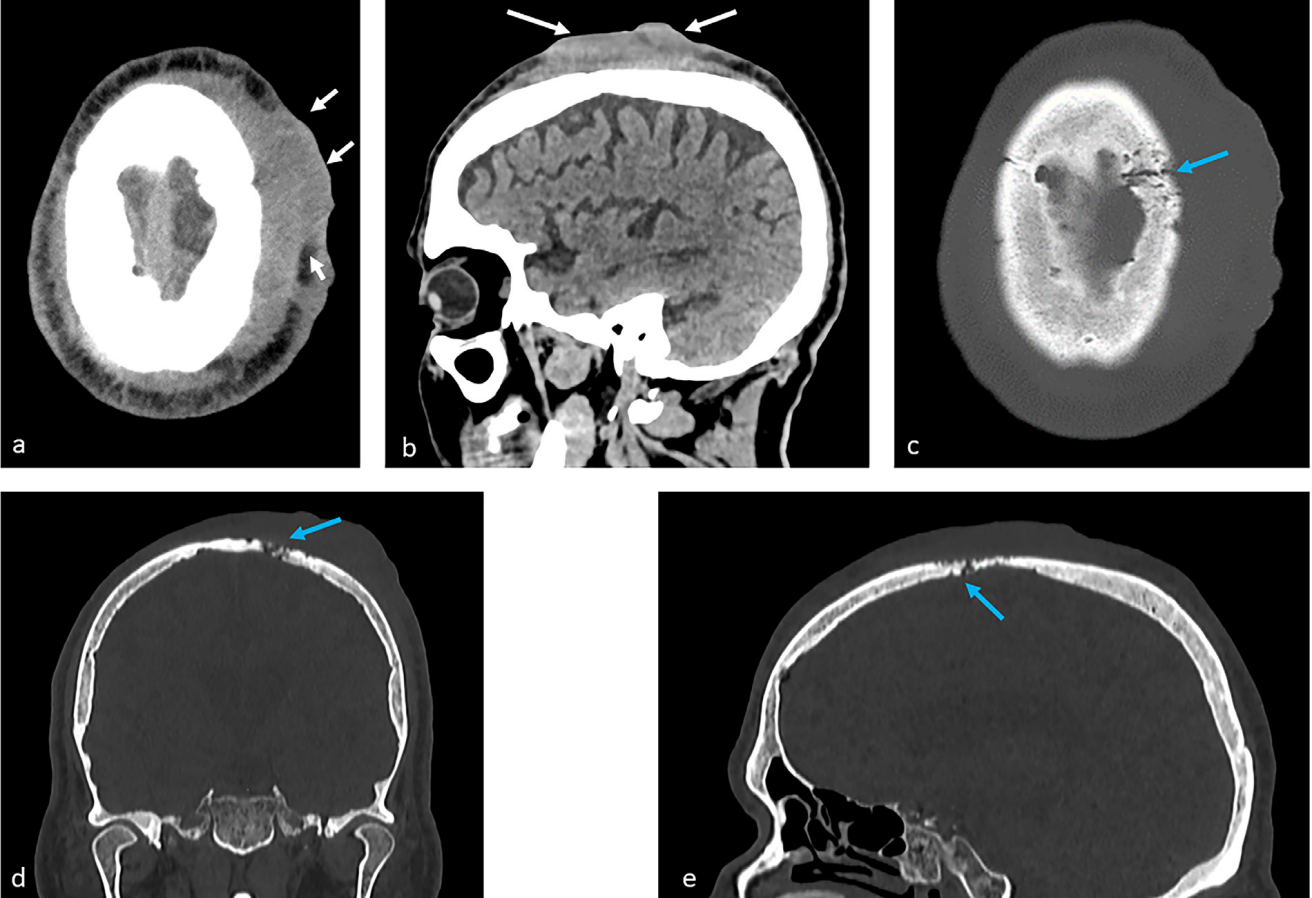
A 77-year-old male with cutaneous angiosarcoma of the scalp. Non–enhanced soft-tissue windowed CT axial (A) and sagittal (B) sections show an irregular exophytic soft tissue lesion arising from the scalp overlying the left frontoparietal bones (white arrows). Bone windowed axial (C), coronal (D) and sagittal (E) sections show associated focal osseous destruction with serpentine osteolytic changes (blue arrows). No intracranial hemorrhage, masses or extension (Color version of the figure is available online.).
